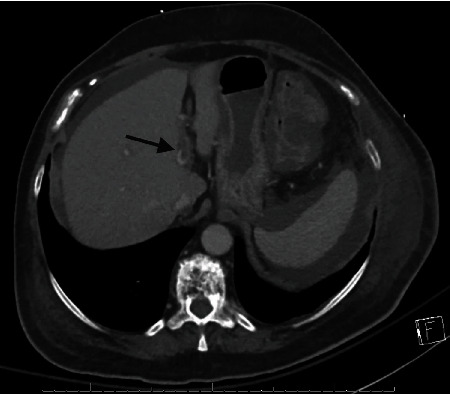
CT abdomen axial view: arrow indicating centrally located filling defect in the left portal vein suggestive of acute thrombus.
